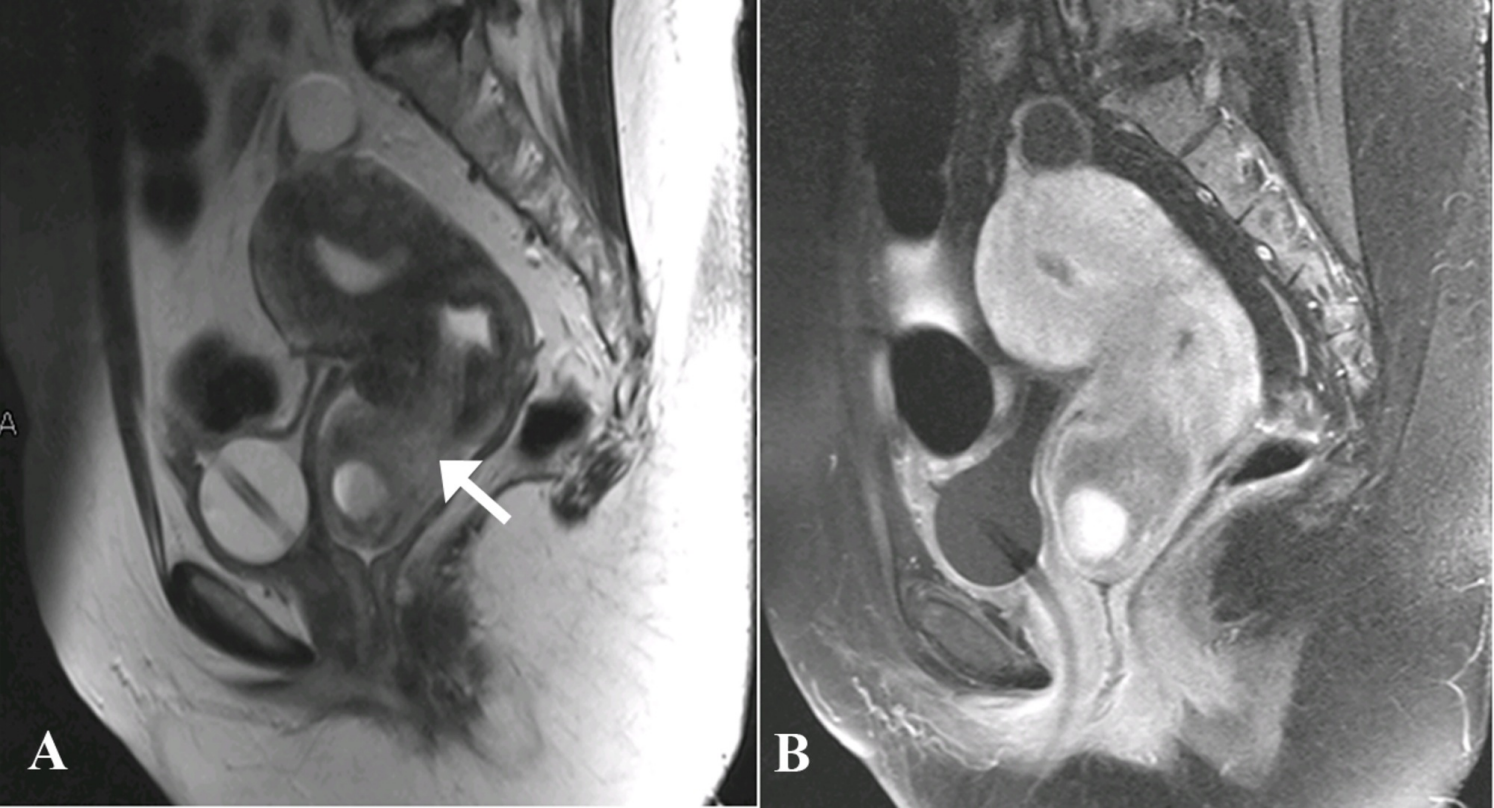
Magnetic resonance images - A) Sagittal T2-weighted and B) Sagittal T1-post contrast. A) A large, well-defined, abnormal signal intensity polypoidal mass lesion (arrow) distending the endocervical canal and extending through the external os lying in the upper one-third of vagina. Cystic spaces noted within the polypoidal lesion in the vagina which are showing fluid-fluid level suggesting hemorrhage. Junctional zone is also ill-defined and diffusely thickened in the uterus measuring approximately 12 mm suggesting adenomyosis. B) The lesion is enhancing heterogeneously. Post-contrast enhancement is relatively less than that of myometrium.
